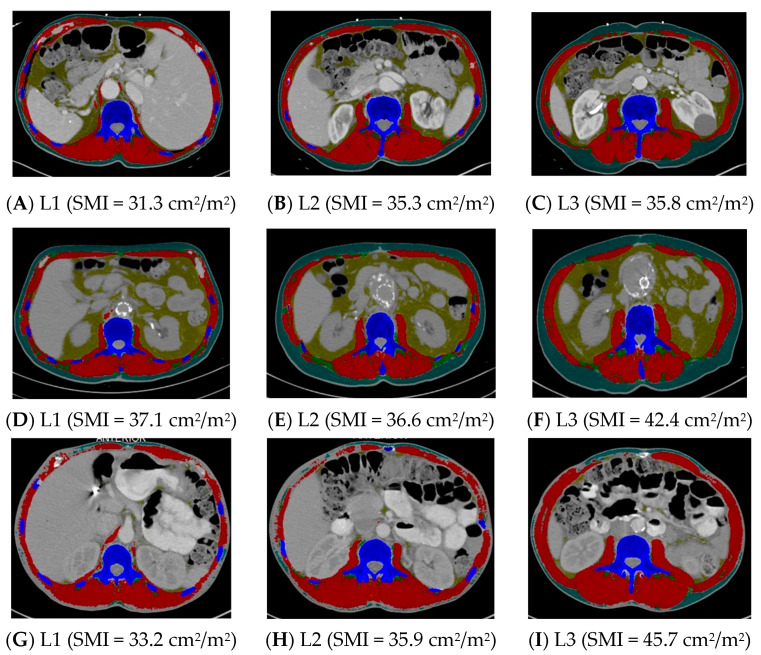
Computed tomography images demonstrating consistency and inconsistency amongst the three vertebral landmarks for the identification of sarcopenia. Images (A–C) consistently identify sarcopenia, whereas images (D–I) inconsistently identify sarcopenia. L1 = first lumbar, L2 = second lumbar, L3 = third lumbar, SMI = skeletal mass index, cm = centimeters, m = meters. Skeletal muscle and adipose tissues were tagged by ABACS+; red= skeletal muscle; yellow= visceral adipose tissue; green= intermuscular adipose tissue; teal= subcutaneous adipose tissue; blue= bone.
